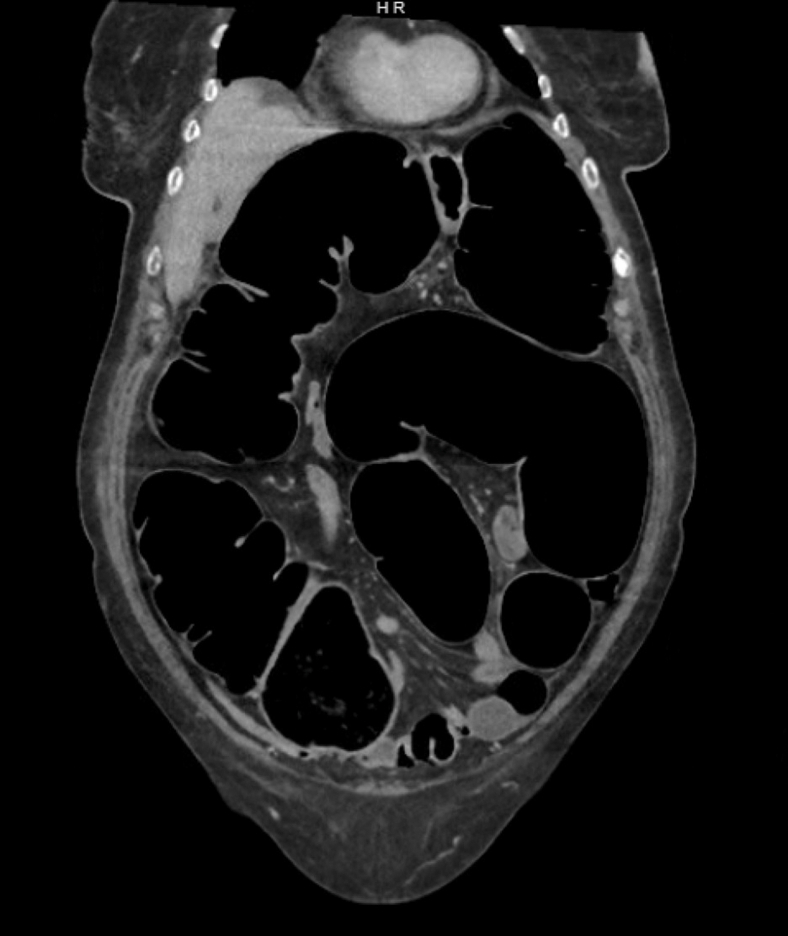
Coronal computed tomography scan revealing diffuse distention of abdominal cavity demonstrating marked dilation of large bowel structures.
